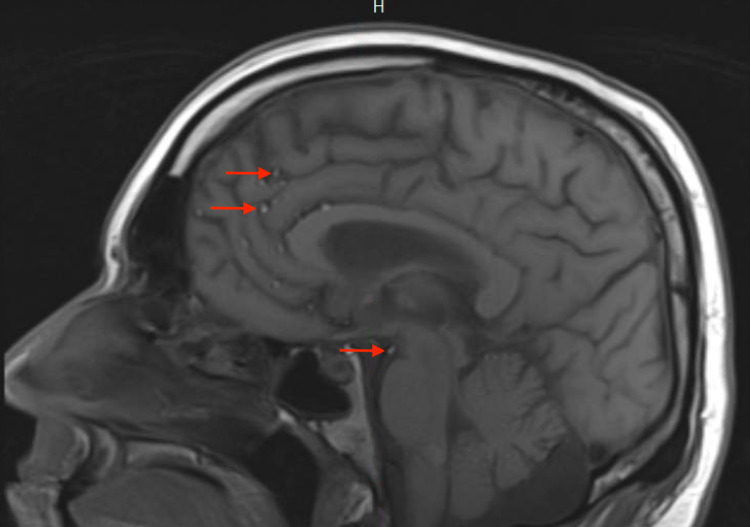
Brain MRI, sagittal T1 Brain MRI T1 shows hyperintensity signal; Red arrows: fat drops in subarachnoid space.
